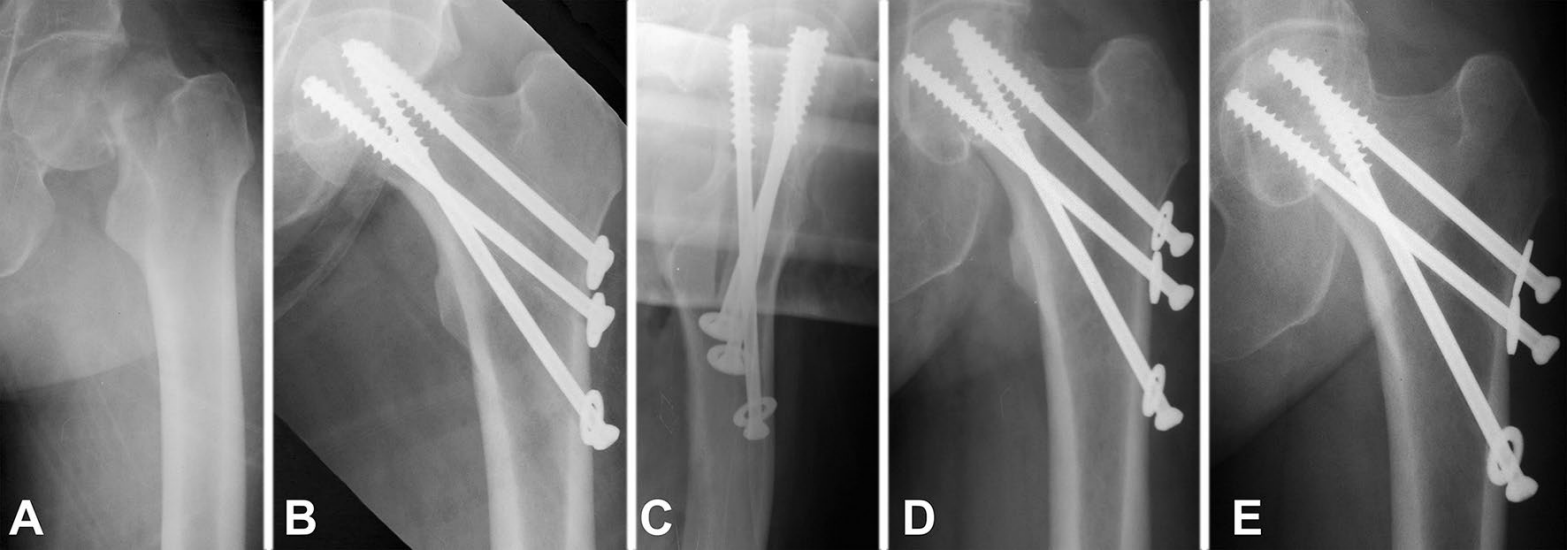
Exemplified X-rays of a 51 yo female patient with bone union (successful healing with fracture consolidation) after BDSF treatment: AP view diagnostics (a), postoperative AP view (b), postoperative lateral view (c), AP view 6-month follow-up (d) and AP view 55-month follow-up (e)
